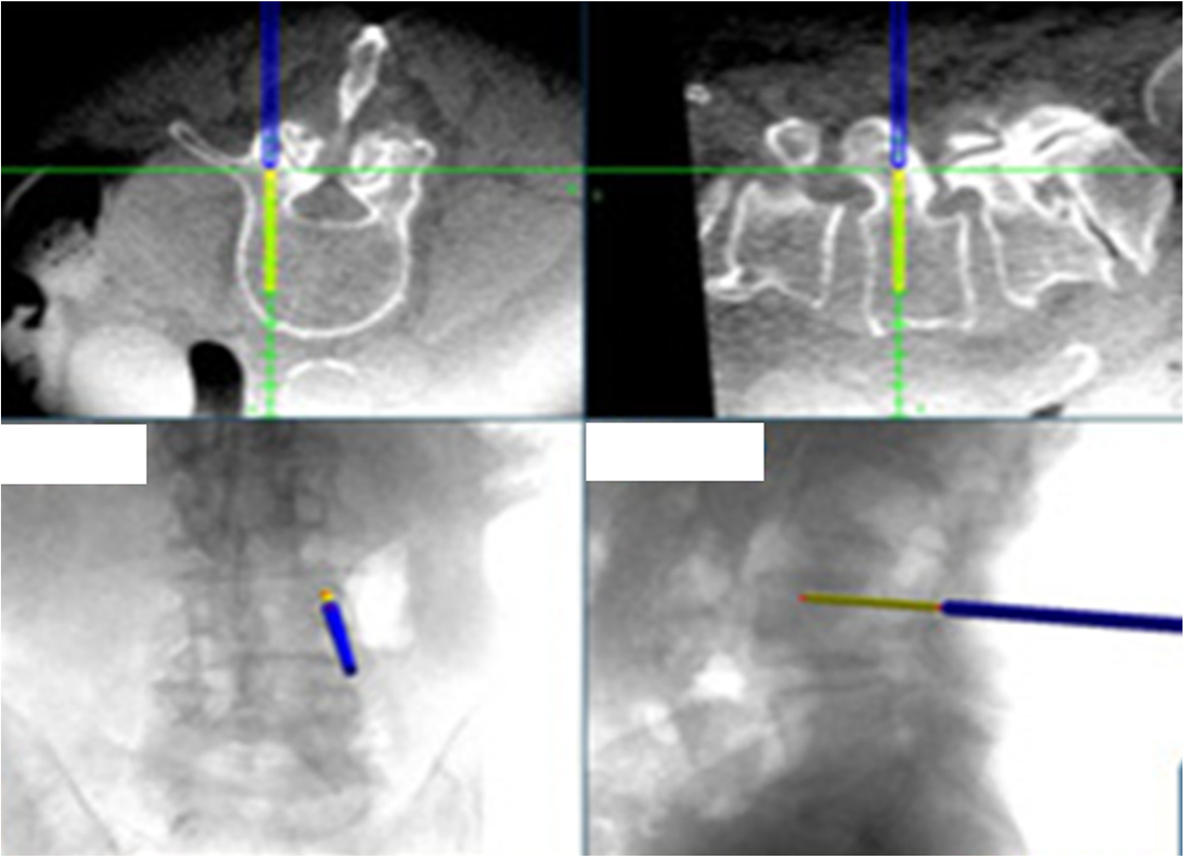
Typical intraoperative CT guidance images
